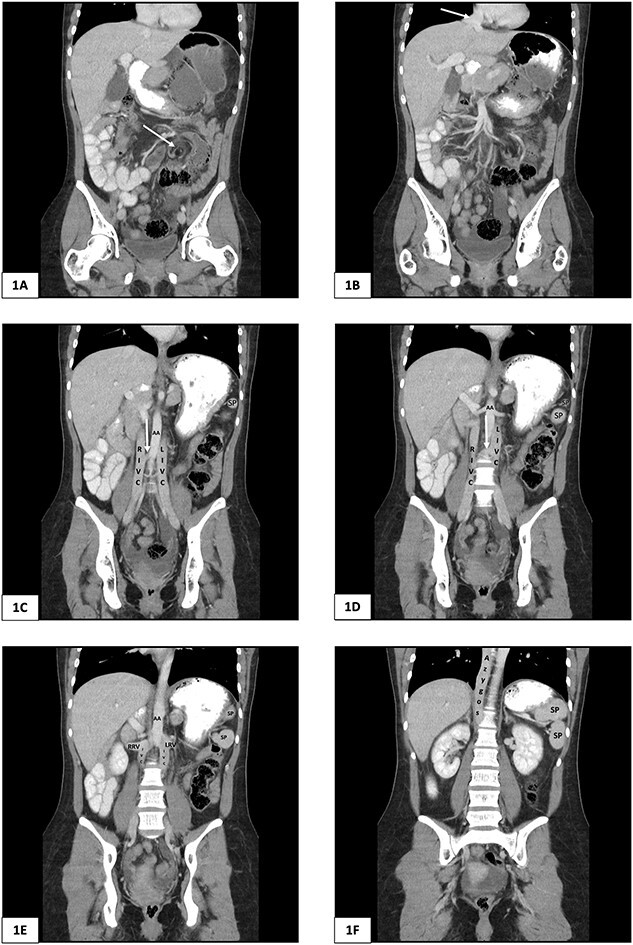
Computed tomography of the abdomen and pelvis of 25-year-old female presenting with caecal volvulus, intestinal malrotation, duplicate inferior vena cava, azygos continuation of the IVC, and fragmentation of the spleen. RIVC, right inferior vena cava; LIVC, left inferior vena cava; RRV, right renal vein; LRV, left renal vein; AA, abdominal aorta; SP, splenic fragment. (A) Arrow indicates mesenteric whirl sign. (B) Arrow indicates suprahepatic IVC draining from right, middle, and left hepatic veins into the right atrium. (C) and (D) Arrow indicates communicating vein from right IVC to left IVC.
